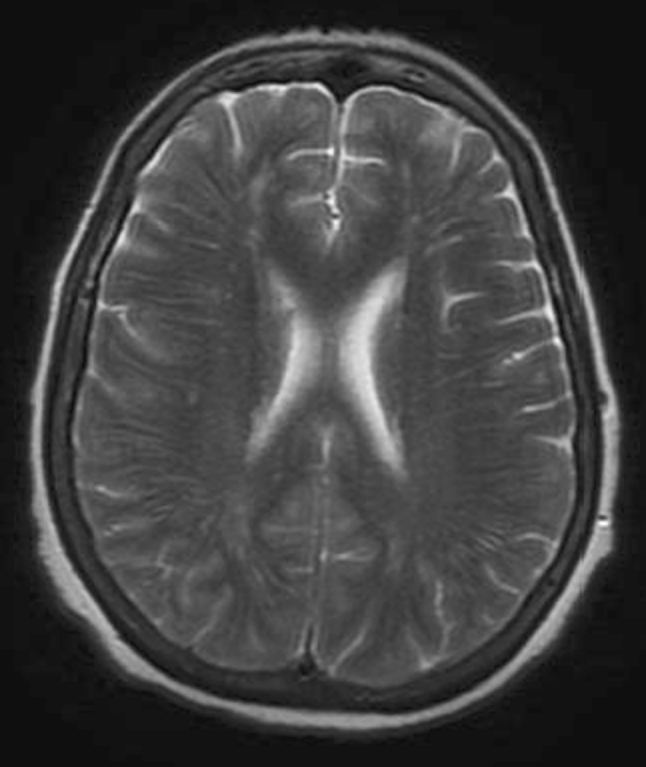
MRI (GE-Philips 1.5T) Patient’s axial T2 weighted normal image
